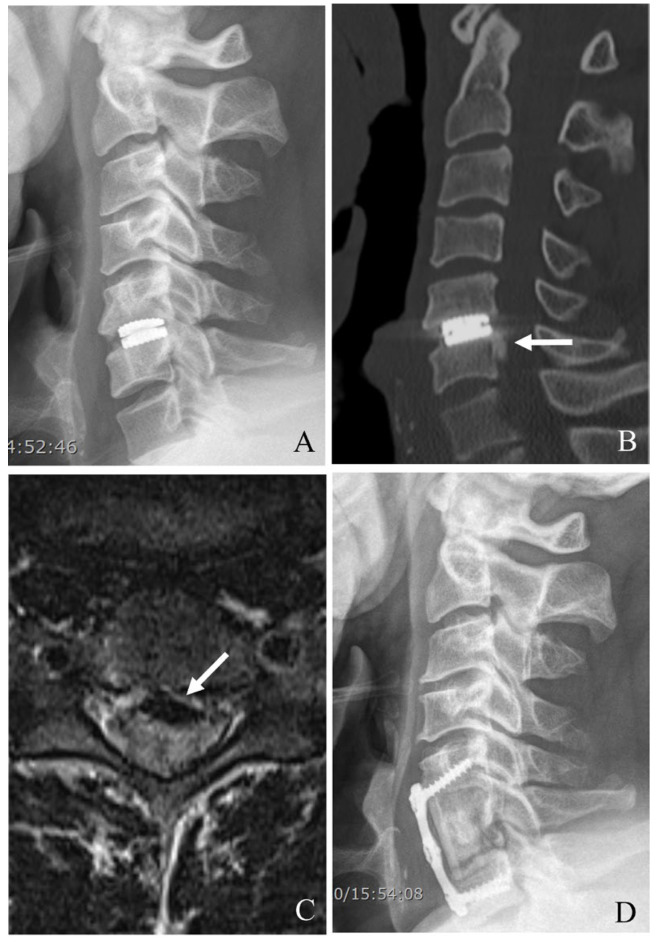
Plain X-rays (A), computed tomography (B), and magnetic resonance image (C) showing cervical disc arthroplasty at C5–6 and ossification of the posterior longitudinal ligament (OPLL) of C6 body (white arrow) causing cord compression. The patient underwent removal of the C5–6 prosthesis and OPLL and anterior cervical corpectomy and fusion (D).
